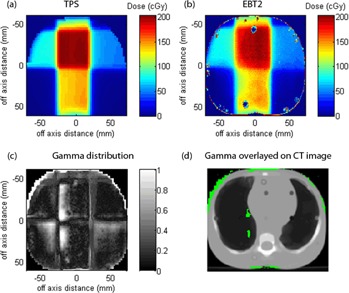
TPS generated dose distribution, (b) EBT2 measurements (average of three measurements) scanned at 75 dpi, (c) gamma distribution (gamma criteria=7%/7mm), and (d) gamma distribution (gamma7%/7mm>1) overlaid on CT image.
